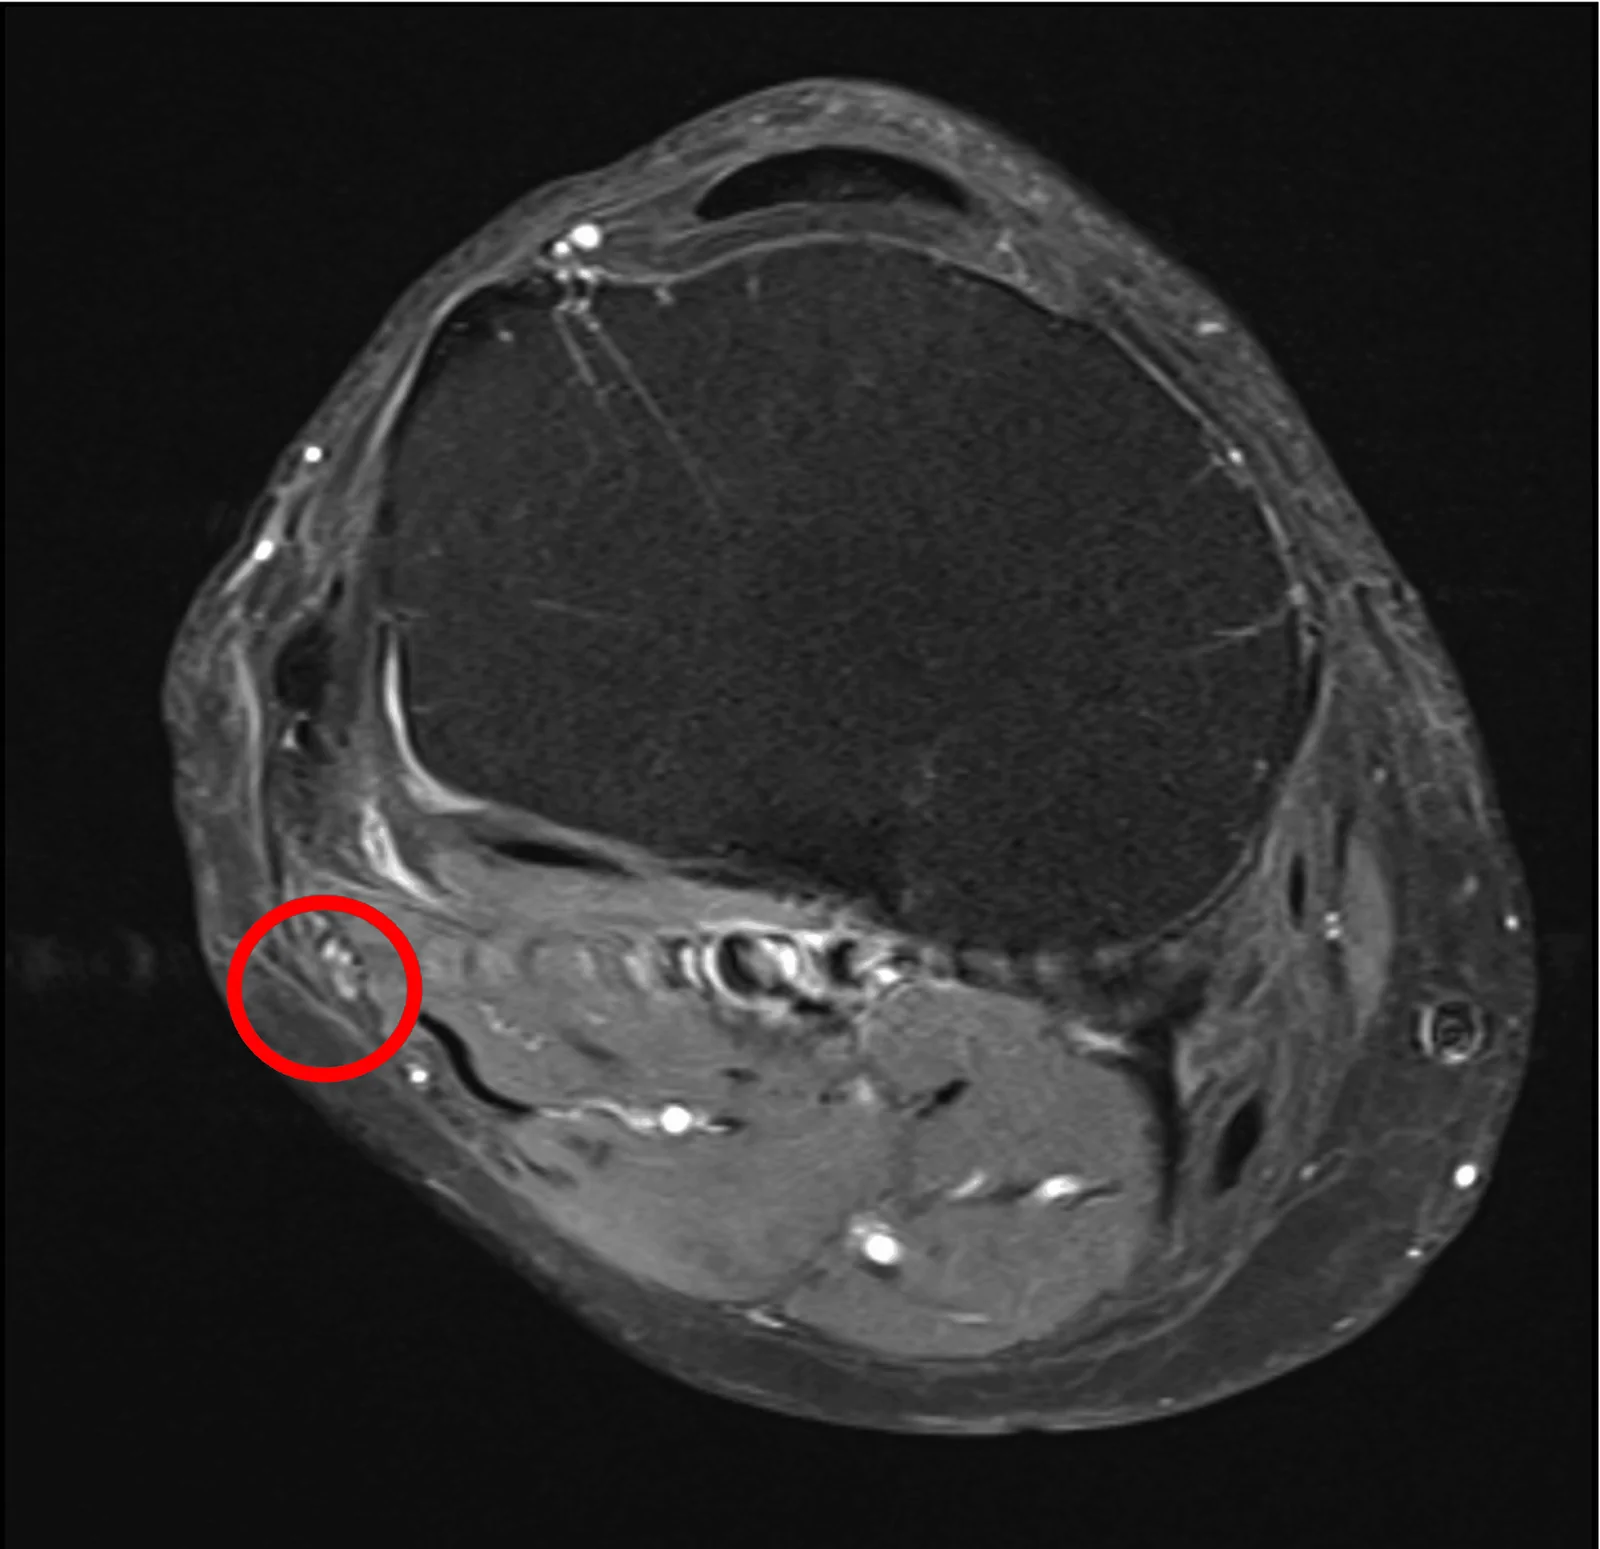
Right knee MRI Axial T1-weighted sequence with contrast and fat saturation—thickening of the peroneal nerve with heterogeneous enhancement of its fibers.
